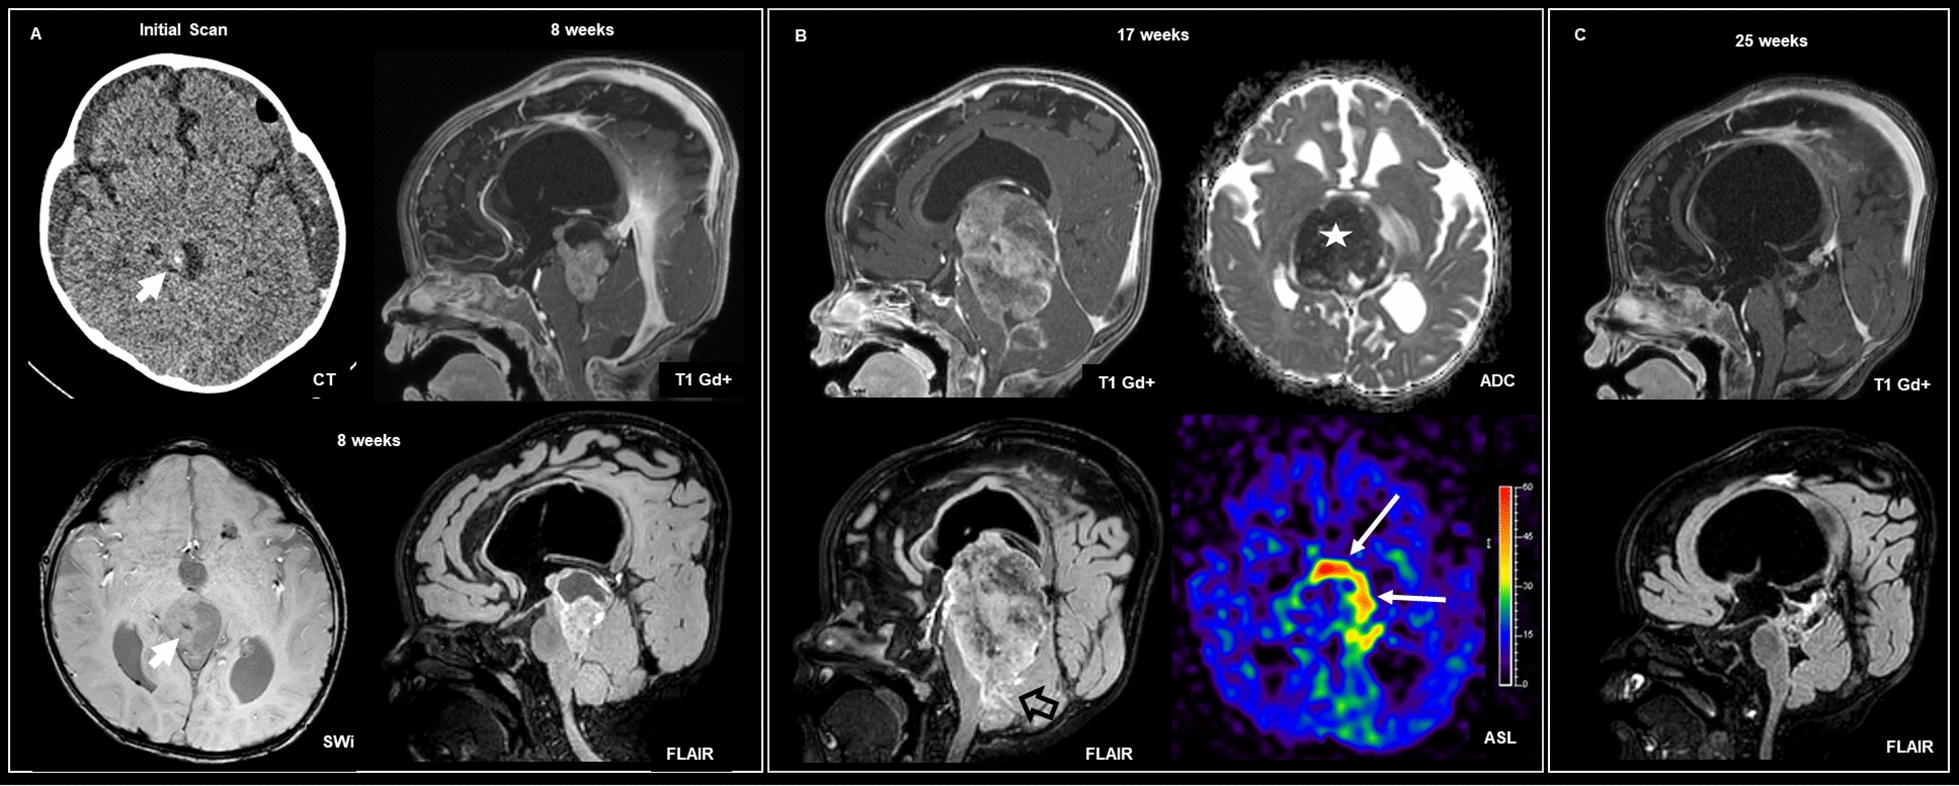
Radiological findings demonstrating response to chemotherapy. Case #4 shown. A A small foci of calcification is depicted on CT scan and susceptibility-weighted imaging MRI (short white arrow). Supratentorial obstructive hydrocephalus and macrocrania are also observed. A, B Heterogeneous tectal and pineal region solid cystic mass, with intense heterogeneous enhancement and rapid overgrowth within two months. B The solid component is highly cellular with marked restriction on ADC map (white star). A rim of increased blood flow on ASL perfusion map is observed in the periphery of the mass (long white arrows), possibly related to neovascularization. Leptomeningeal enhancement is also observed (open black arrow). C Significant shrinkage of the tectal and pineal region mass after chemotherapy was observed, with nearly complete resolution of the intracranial leptomeningeal enhancement
